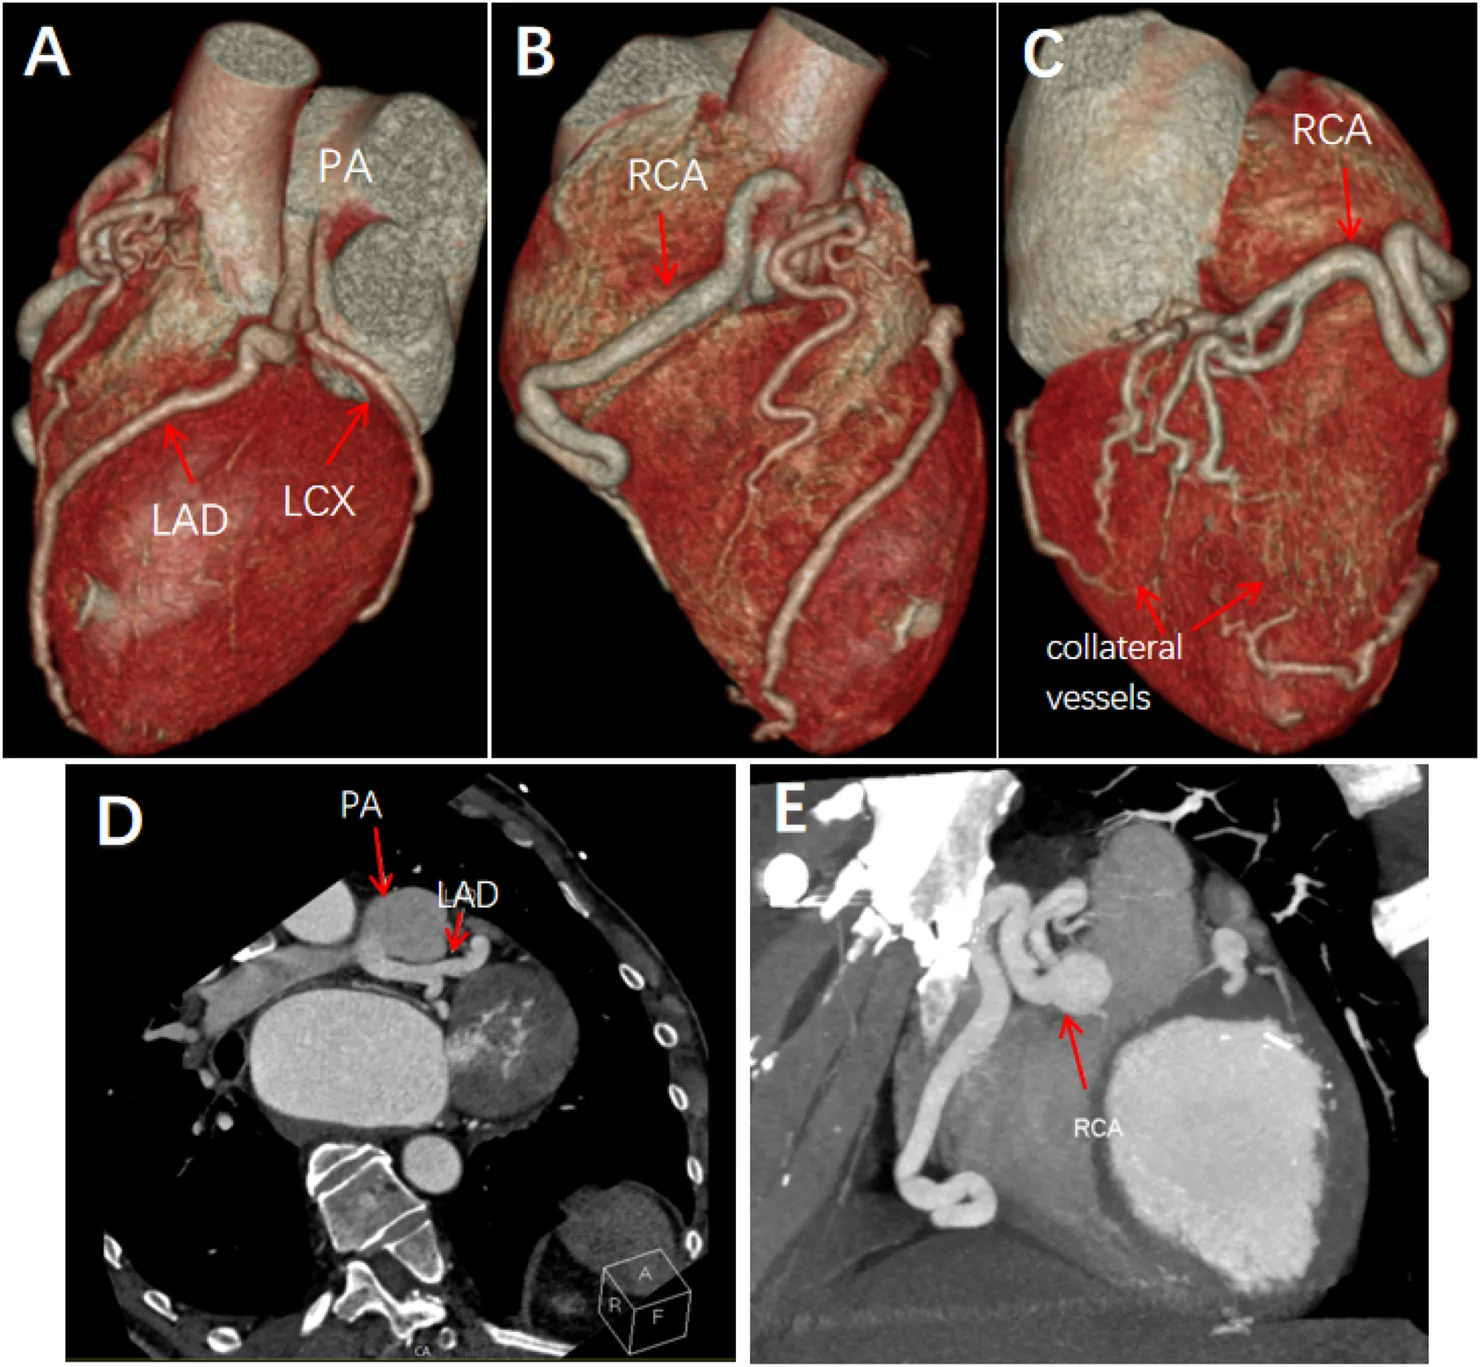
Volume-rendering (VR) images and slice views of coronary computed tomography angiography. (A) VR shows that the LCA originates from the PA. (B) VR shows that the RCA is tortuous and dilated. (C) VR shows multiple small tortuous collateral vessels formed between the LAD and the RCA. (D) An oblique sagittal view shows that the left coronary artery abnormally originates from the pulmonary artery. (E) A short-axis view shows that the RCA is tortuous and dilated. LCA, left coronary artery; PA, pulmonary artery; RCA, right coronary artery; LAD, left anterior descending branch; LCX, left circumflex branch.
